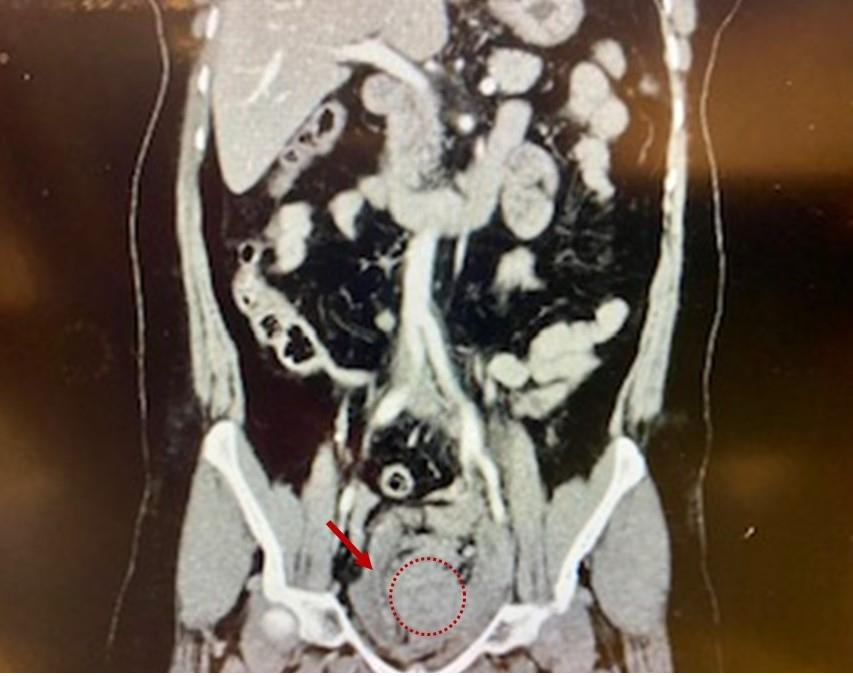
Initial computed tomography of the abdomen and pelvis, with pelvic hematoma compressing the bladder and ovaries. *Red arrow*: compressed and displaced bladder. *Dotted circle*: forming pelvic hematoma.
